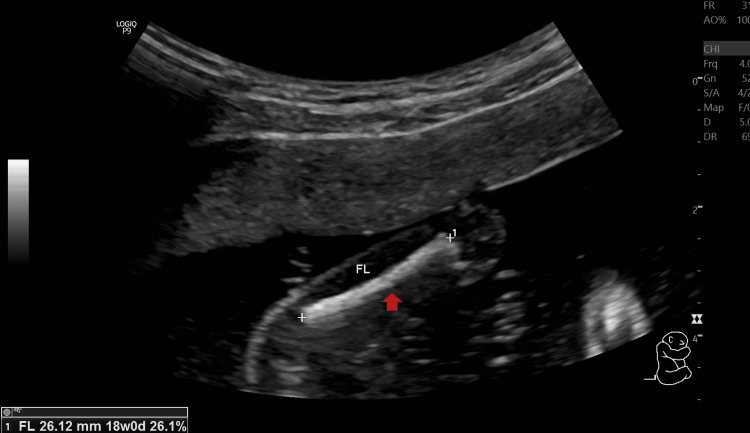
B-mode ultrasonography image showing the femur length of the fetus. The arrow (⬆) points to the femur of the fetus. The measurement of the femur can be read in the bottom left-hand corner of the image. Femur length: 26.12 mm at 18.0 weeks.
